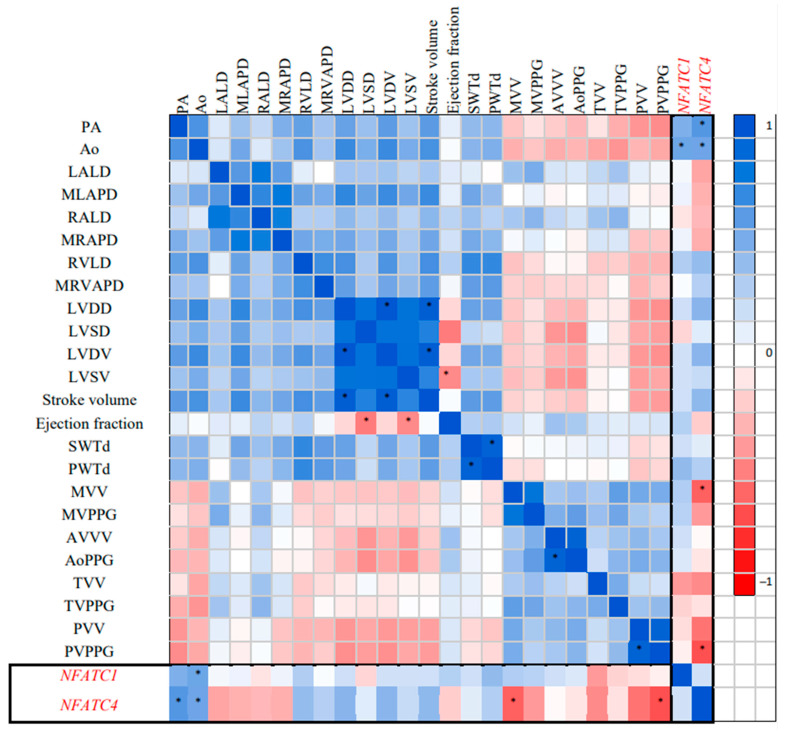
Gradient pattern of correlation matrix for NFATC1 and NFATC4 expression with Doppler echocardiographic parameters in children with BAV (*n* = 47). * Correlations marked at *p* < 0.05.
